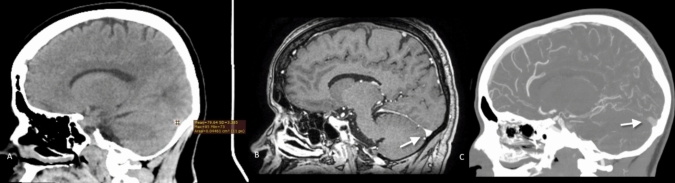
A–C The torcula of 30 year-old female appeared hyperattenuating on non-contrast computed tomography with an average attenuation of 79 HU (A) and was interpreted as thrombosed. However, contrast-enhanced CT in sagittal (B) and CT venography in sagittal (C) reconstruction showed patency of the torcula. This false positive result is likely attributed to the patient’s elevated hematocrit level of 50%
